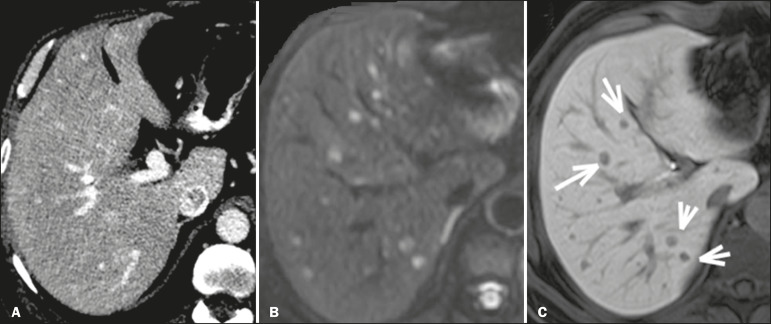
Arterial phase CT scan (A), DWI scan with a b value of 800 s/mm^2^ (B), and 20-min hepatobiliary phase MRI scan (C). The CT scan shows some sparse hypervascular nodules in the liver parenchyma. However, a close look at the images obtained with MRI using hepatobiliary-specific contrast, analyzing the DWI scan together with the hepatobiliary phase scan (arrows in C), reveals that there were multiple liver nodules.
